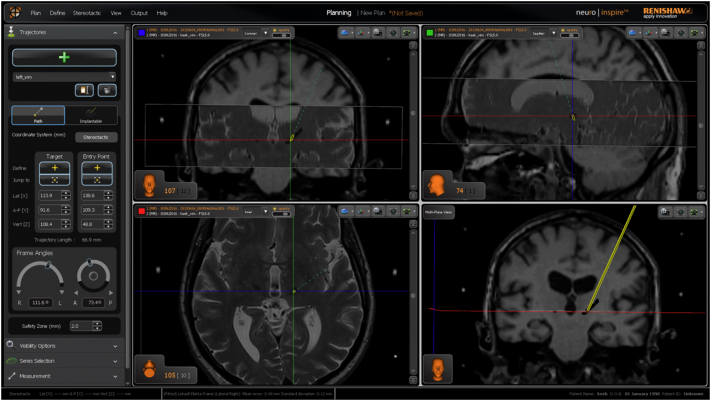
Left VIM DBS planning using Neuroinspire surgical planning software using preoperative stereotactic T2-weighted slab registered to MPRAGE T1 NIfTY volume with dentate-thalamic clusters punched-out
